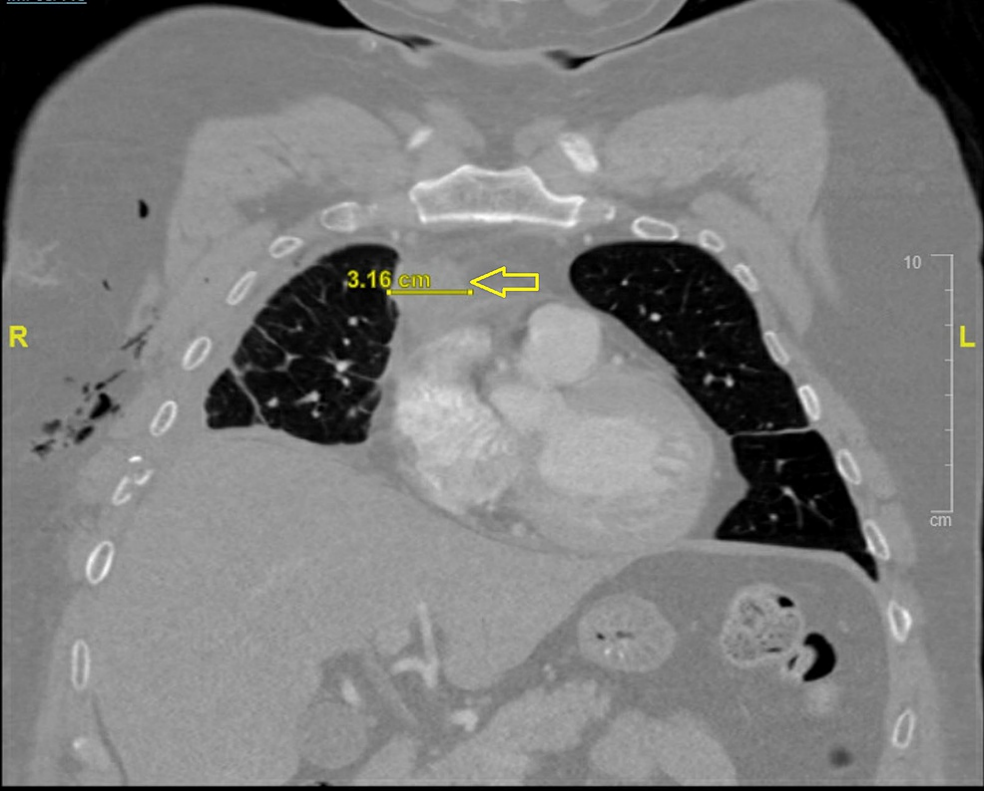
CT chest with contrast showing anterior mediastinal mass (yellow mass) measuring 3.16 cm transverse diameter
